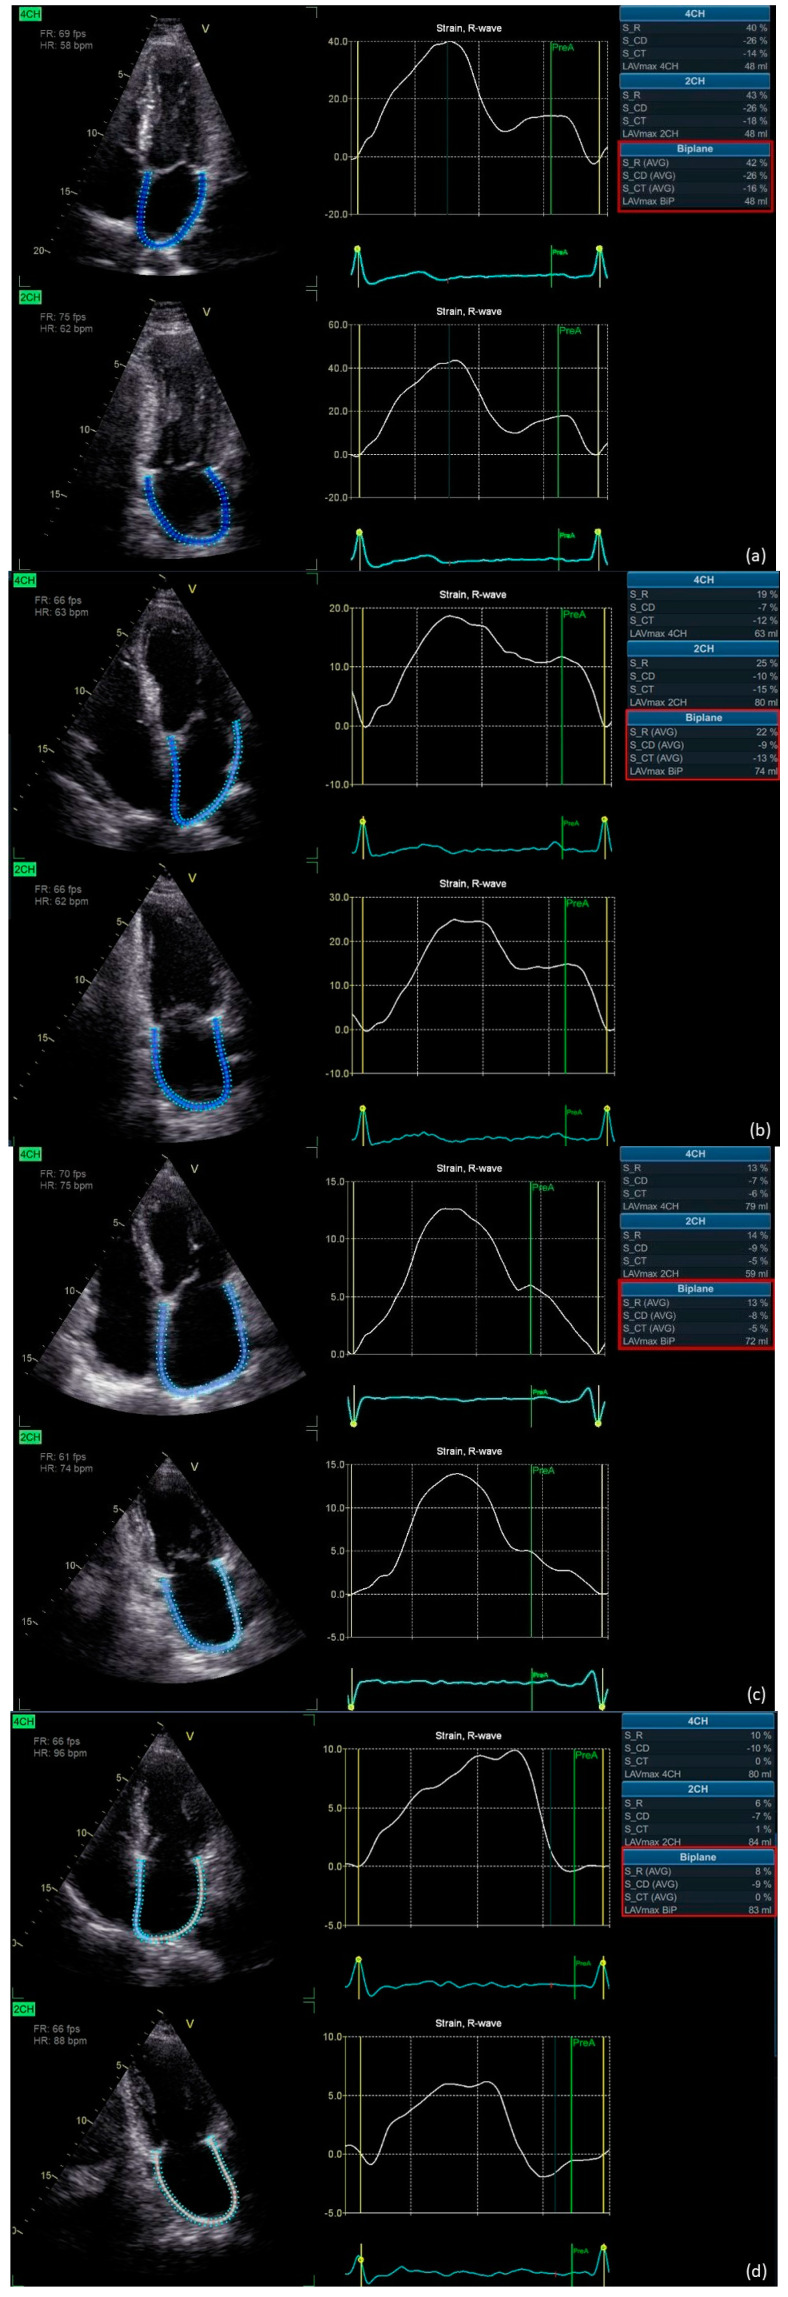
Examples of left atrial strain (LAS) values: (a) normal LAS in a patient with sinus rhythm during echocardiography, (b) slightly decreased LAS in a patient with sinus rhythm during echocardiography, (c) significantly decreased LAS in a patient with sinus rhythm during echocardiography, and (d) LAS in a patient with atrial fibrillation during echocardiography. Abbreviations: LAVmax 2CH, maximum left atrial volume in two-chamber apical view; LAVmax 4CH, maximum left atrial volume in four-chamber apical view; LAVmax BIP, maximum biplane left atrial volume; S_CD, left atrial conduit strain; S_CT, left atrial contraction strain; S_R, left atrial reservoir strain.
